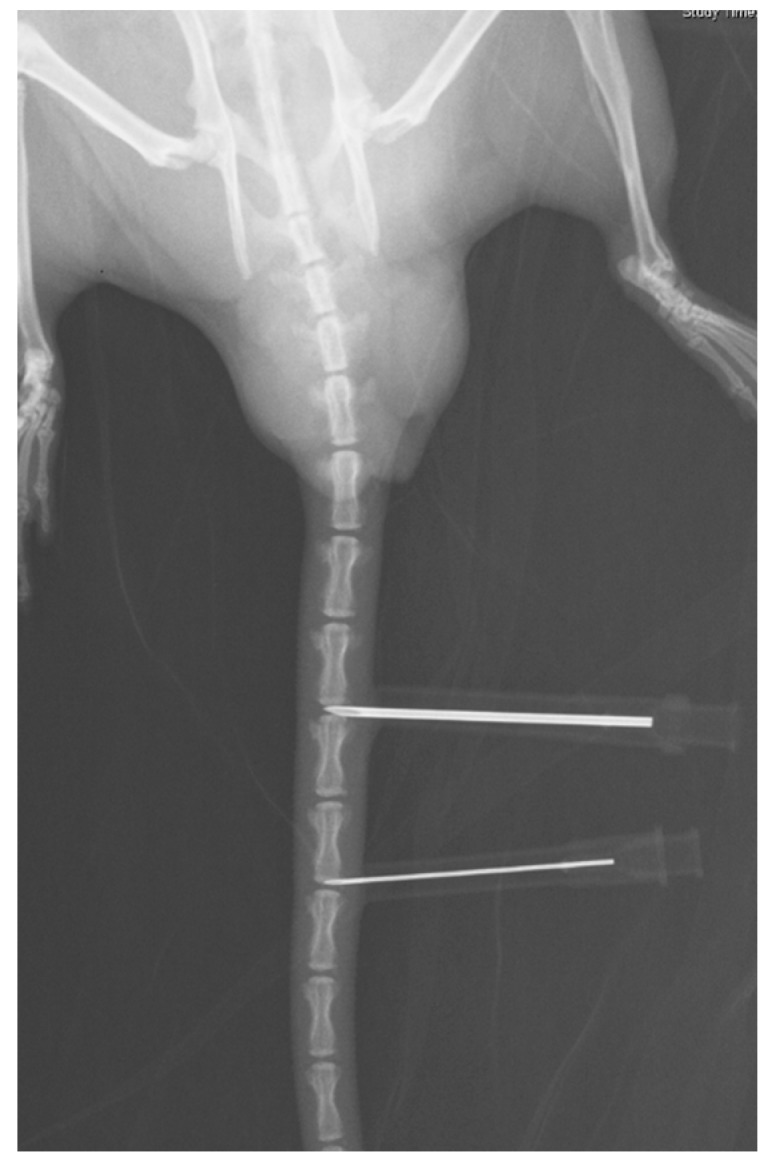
A radiograph demonstrating two needles puncturing Co6-7 and Co8-9 discs.
